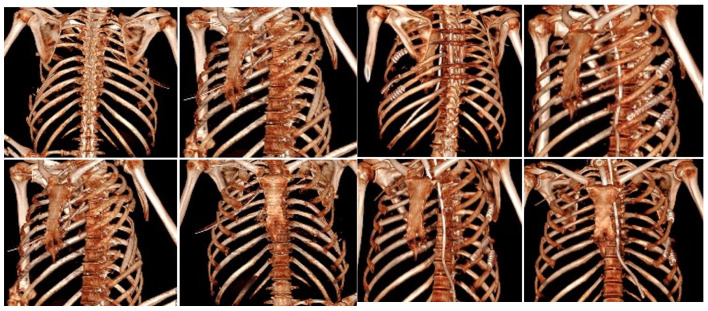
Preoperative CT 3D reconstruction can identify displaced fractures from the third to the eighth left ribs. The patient also experienced a chest wall laceration with lung tissue herniation. The postoperative CT scan shows correction of the fractures through the placement of grafts, titanium plates, and screws.
